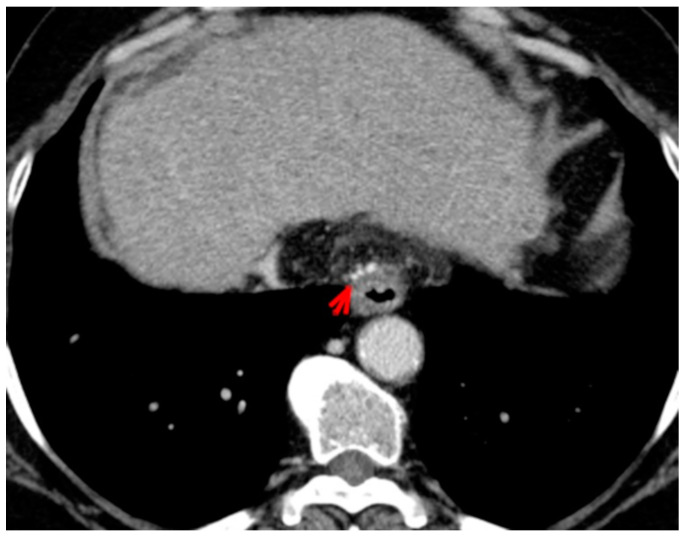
Axial contrast-enhanced CT shows enlarged paraoesophageal varices (red arrow).
